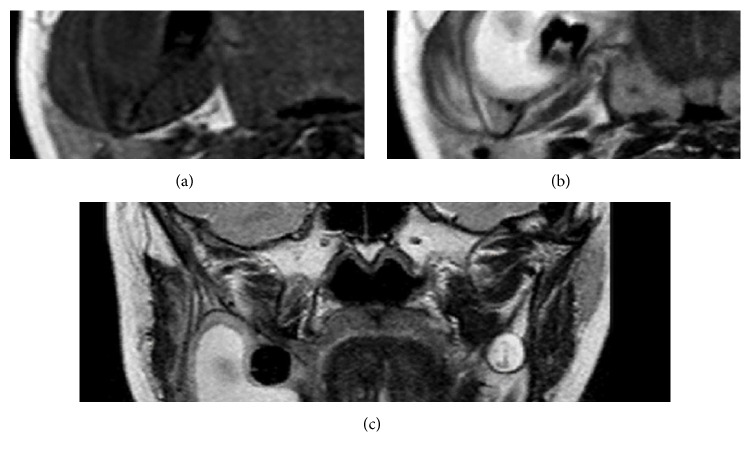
MR images showing T1 axial view (a), T2 axial view (b), and T2 coronal view (c).
